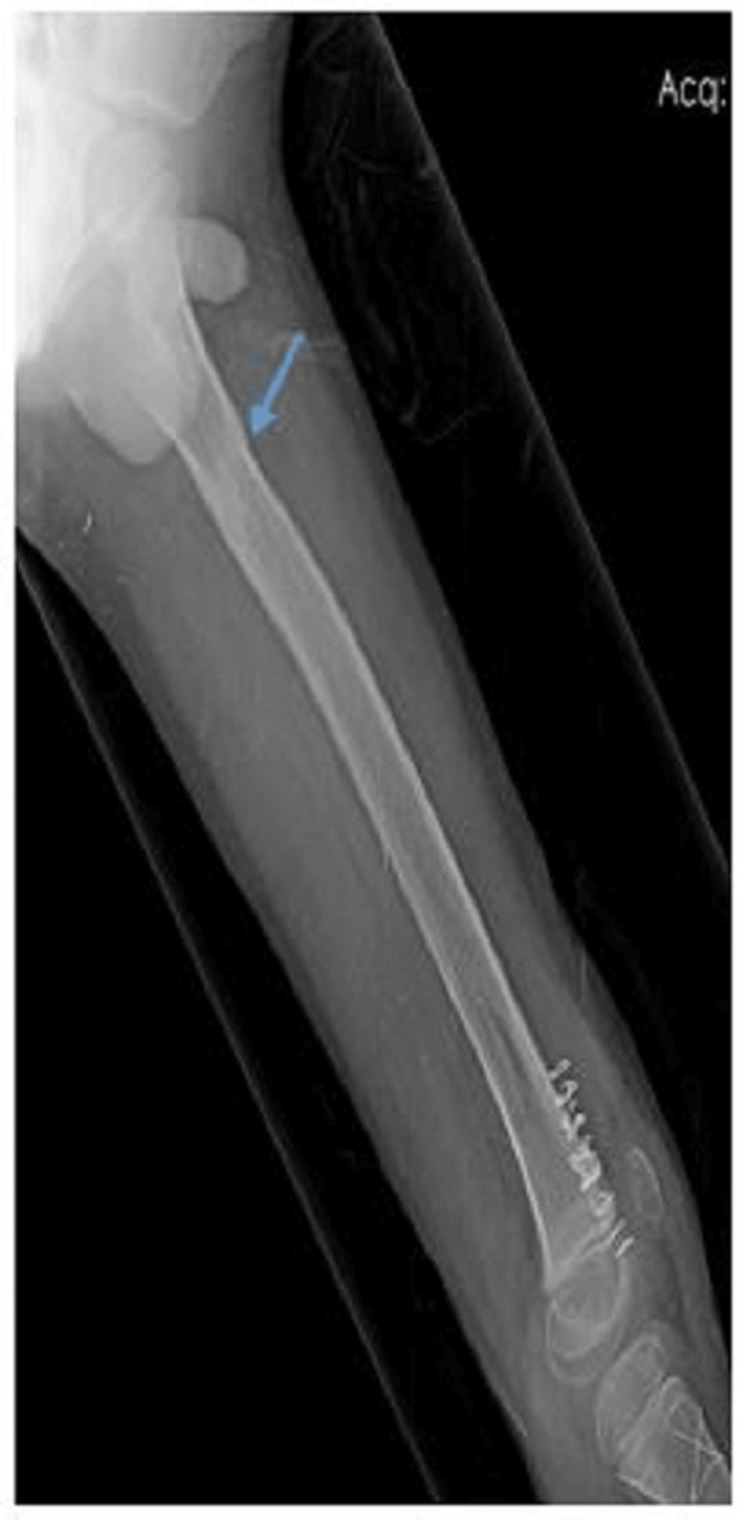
Lateral radiograph of a nine-year-old male who underwent implant removal of TENS after fracture union (blue arrow) TENS: titanium elastic nailing system
